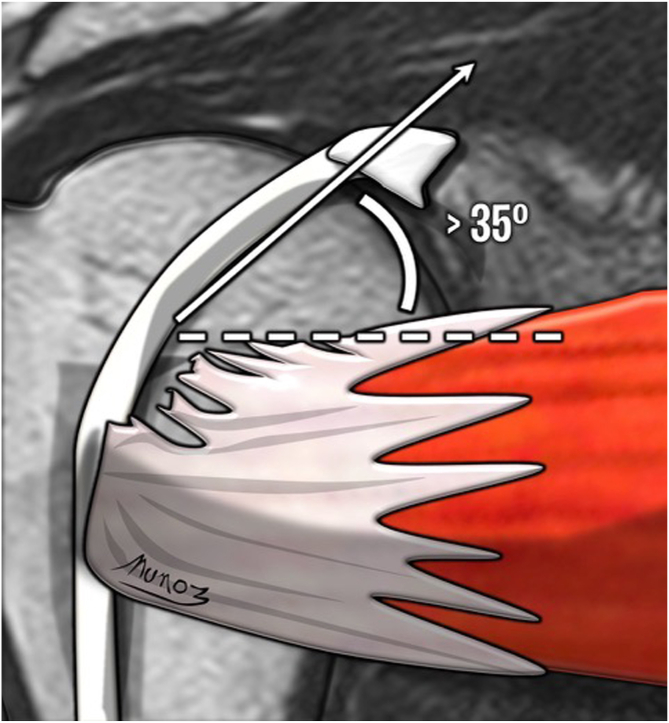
LHBT angle sign is shown in a coronal view of a T1-weighted right shoulder MRI. The angle is measured between the intra-articular portion of the LHBT and the superior edge of the SSC tendon. An angle >35° is considered a positive sign. (LHBT, long head biceps tendon; MRI, magnetic resonance imaging; SSC, subscapularis tendon.)
